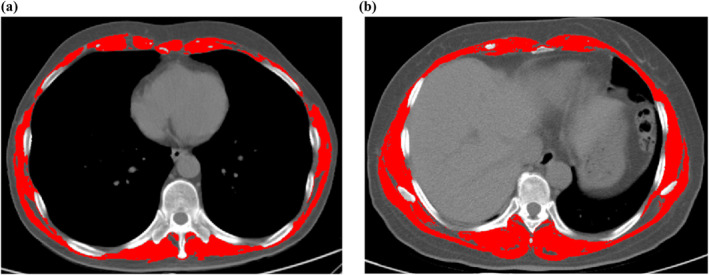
The red shaded part in the computed tomography image represents the area of skeletal muscle. (a) T10 skeletal muscle index (SMI) = 20.58 cm^2^/m^2^. (b) T10 SMI = 40.99 cm^2^/m^2^.
